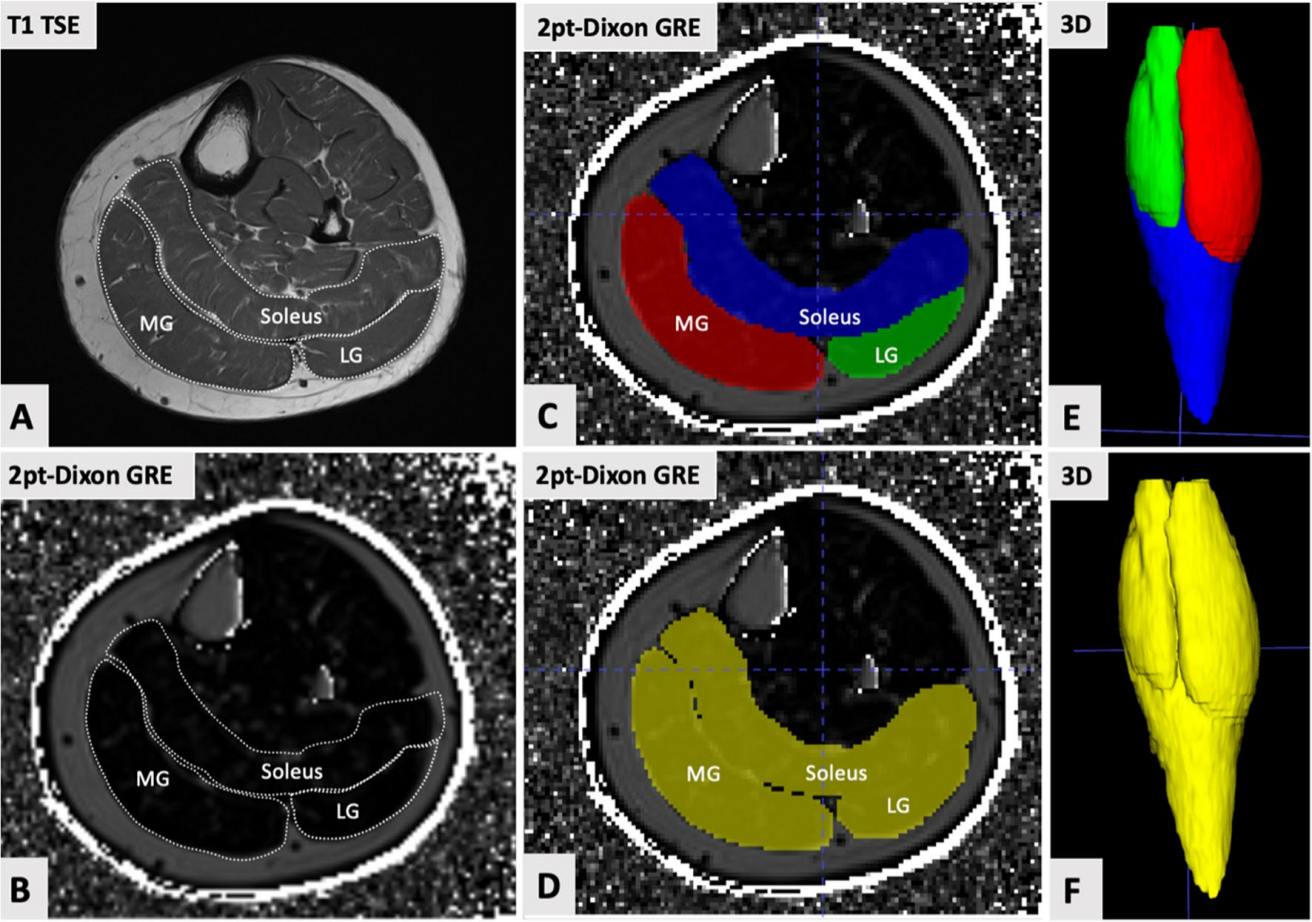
Quantitative volumetric assessment of calf muscle fat content (MFC) using the example of a 68-year-old female (left side). Outlining of calf muscles was done in conjunction with the corresponding transversal T1-weighted sequence (A). The reconstructed two-point Dixon gradient-echo (GRE) MR images were uploaded into ITK-Snap (B). Next, the medial gastrocnemius (MG), lateral gastrocnemius (LG), and soleus muscles were manually outlined on all slices (C). After that, automated three-dimensional (3D) reconstructions were generated with an output of the mean volumetric fat fraction (FF) and whole muscle volume (WMV) for each muscle separately. In this case, the MG muscle demonstrated a FF of 10.3% (WMV = 145.8 cm^3^), the LG muscle of 8.6% (WMV of 85.3 cm^3^), and the soleus muscle of 10.7% (WMV = 211.2 cm^3^). These segmentations (MG, LG, and soleus muscles) were summed up to determine the FF and WMV of the triceps surae muscle (D). In this example, the FF of the triceps surae muscle was calculated with 10.2% (WMV = 442.3cm^3^). E, F The corresponding 3D reconstruction images
